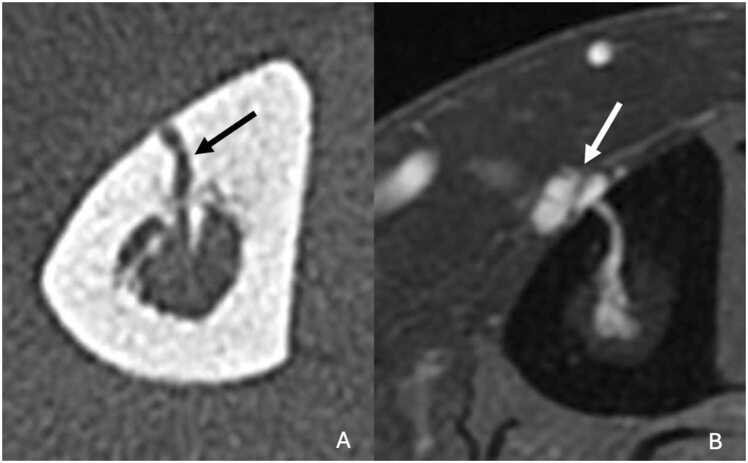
Intraosseous varicose vein in a 64-year-old woman with a history of surgery for bilateral varicose veins of lower limbs, presenting chronic pain in the left leg for several years. A. CT image in the axial plane highlighting this serpiginous vessel passing through the tibial cortex (black arrow). B. T1-weighted fat-suppressed and contrast enhanced MR image in the axial plane showing this dilated intraosseous vessel communicating with varicose vein touching the tibial cortex (white arrow). Interventional treatment by sclerotherapy has reduced symptoms.
